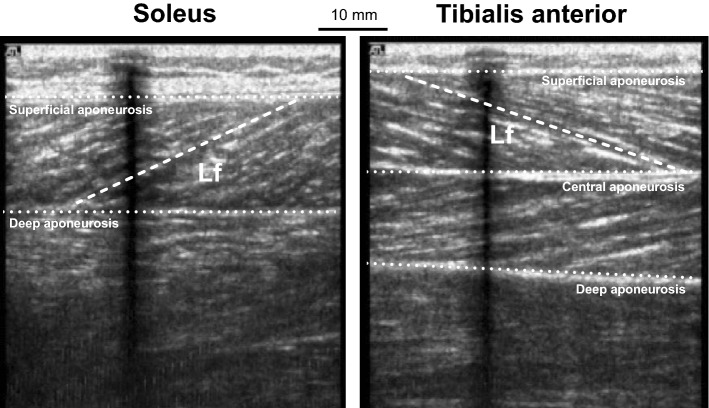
An example of ultrasound sagittal plane scans. Images were taken at anatomical zero and show the fascicle length (Lf) measured from the visible insertion of the fibre between the deep and superficial aponeurosis in soleus (left panel), and from central to the superficial aponeurosis tibialis anterior (right panel). The shadow in the images represents the echo-absorptive marker used to ensure no movement between the skin and the probe occurred throughout ankle movement
